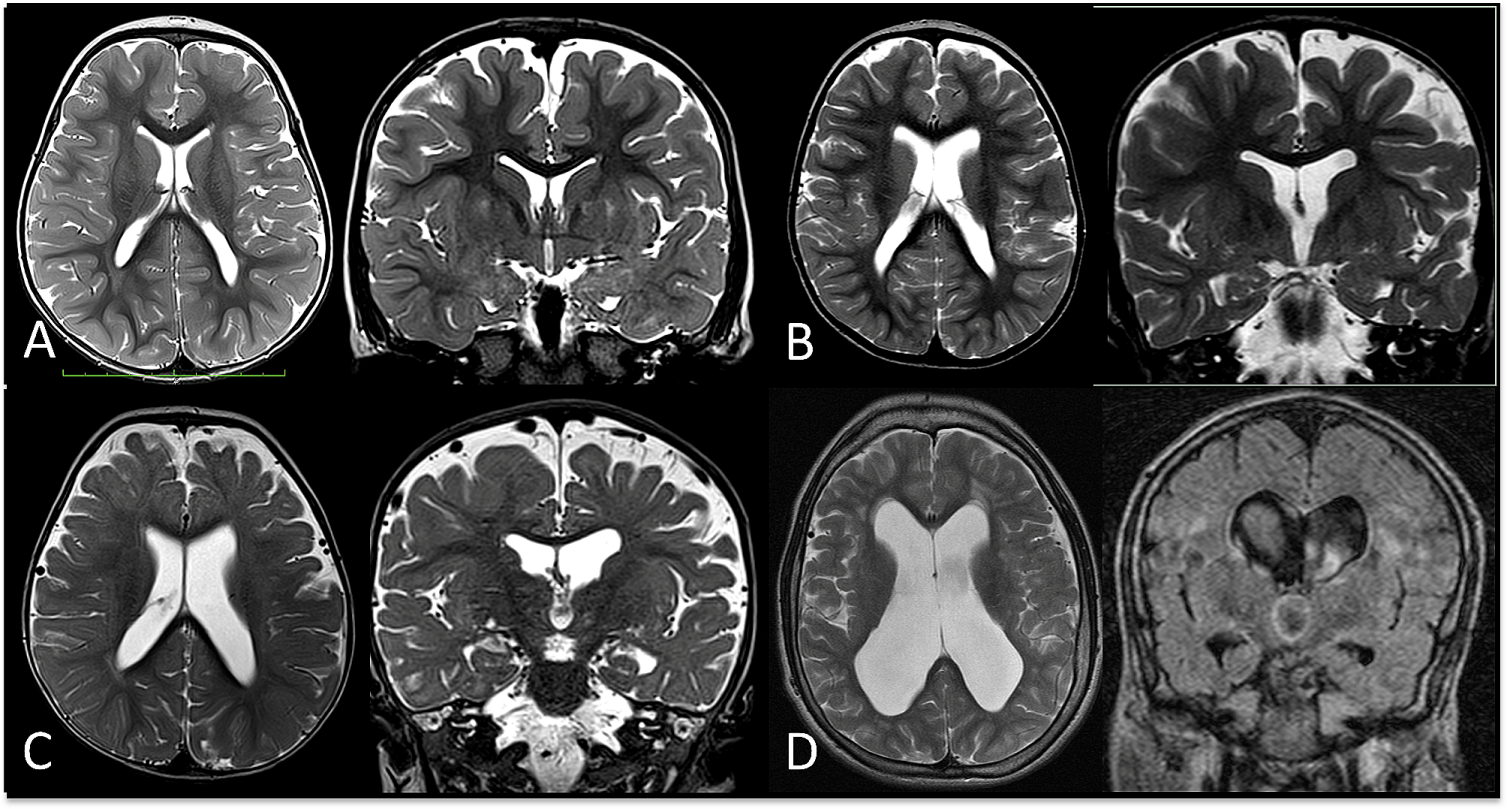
Brain Trophism and Ventricular System. A, Regular volume of the ventricular system with a slight widening of the peri-cerebral subarachnoid spaces (Case 18, 2 years old); B, mild enlargement of the lateral ventricles and of the peri-cerebral subarachnoid spaces (Case 22, 4 years old); C, more prominent ventriculomegaly and dilatation of the peri-cerebral subarachnoid spaces (Case 11, 1 year old); D, communicating hydrocephalus, with signs of hyperdynamic flow (flow-related artifact on coronal FLAIR on the right) (Case 31, 17 years old)
